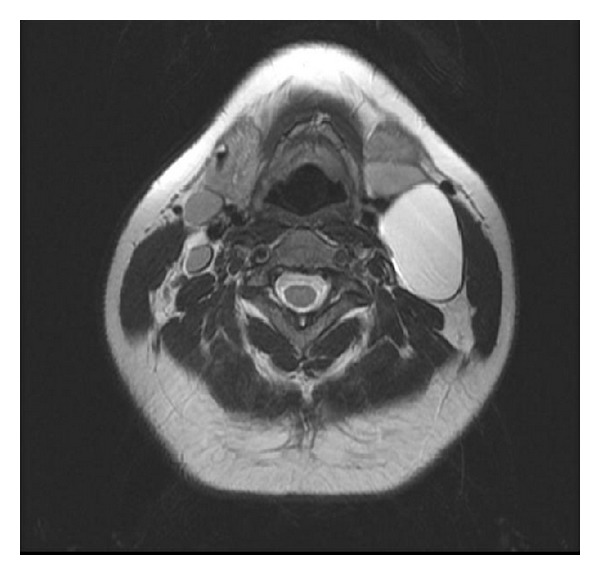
MRI-axial view showing the cystic lesion with high T2 signal.
